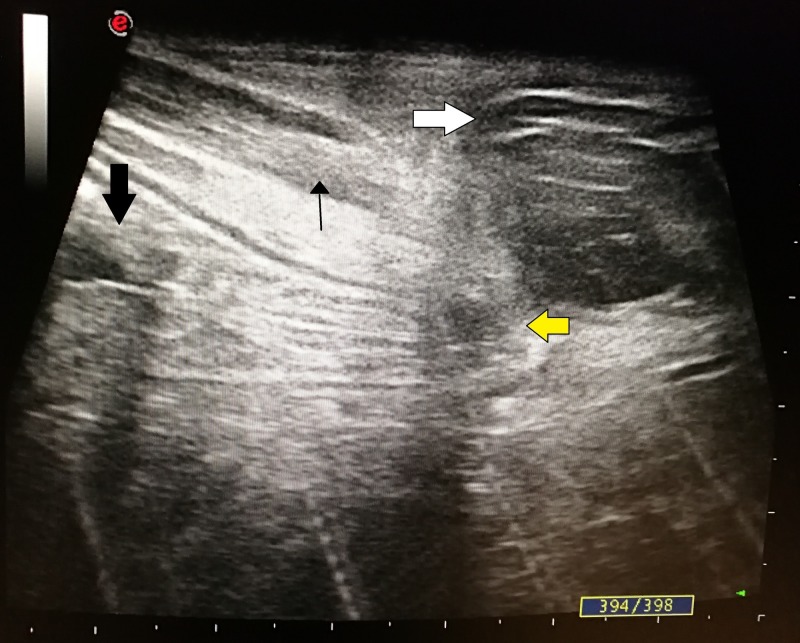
Ultrasonography (USG) image showing the internal bumper (white arrow) seen within the rectus abdominis muscle (thin black arrow). Thick black arrow showing the gastric wall, and yellow arrow showing the tract through which the internal bumper had migrated.
